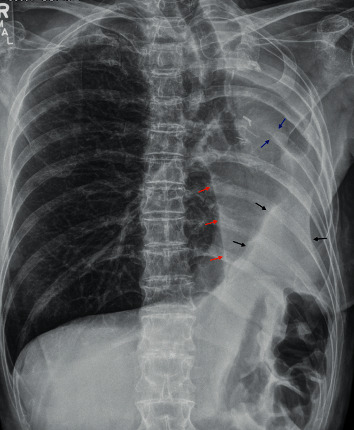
Anteroposterior chest radiograph demonstrates pneumonectomy surgical clips, suture, and left thoracostomy (blue arrows) with many secondary signs of left hemithorax volume loss including leftward tracheal shift, leftward shifting of the azygoesophageal recess (red arrows), upward tenting of the left hemidiaphragm (black arrows), and ipsilateral intercostal space narrowing.
